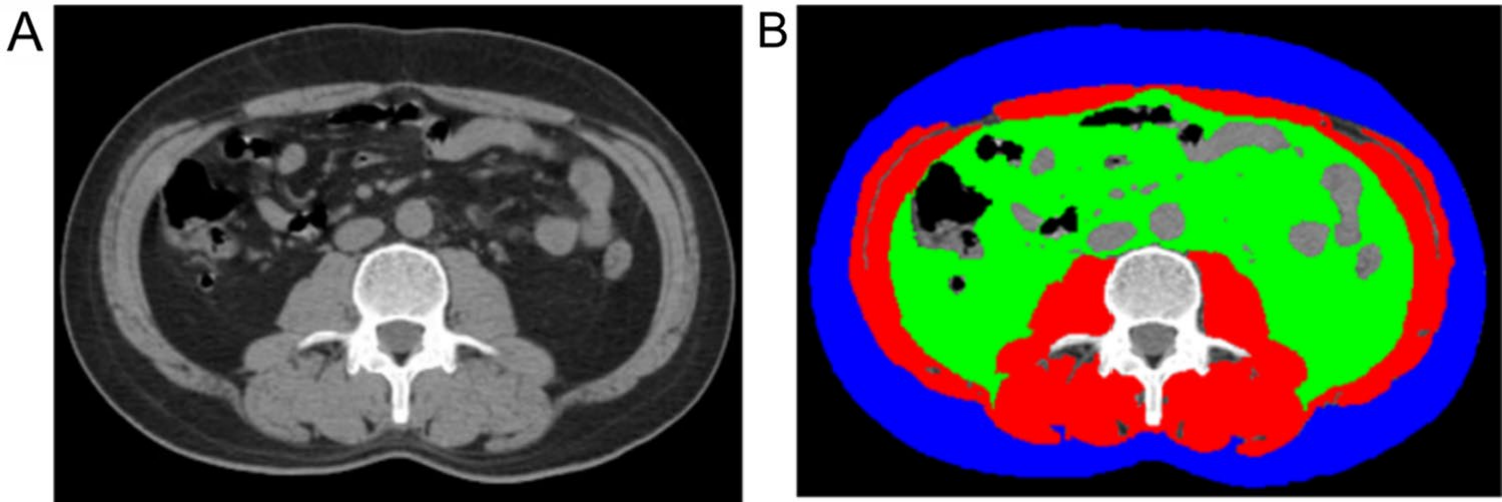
Example of threshold-based delineation of fat and skeletal muscle parameters on abdominal CT images at the 3rd lumbar vertebral level (blue, subcutaneous fat area; green, abdominal visceral fat area; red, abdominal wall and lumbar muscle area)
